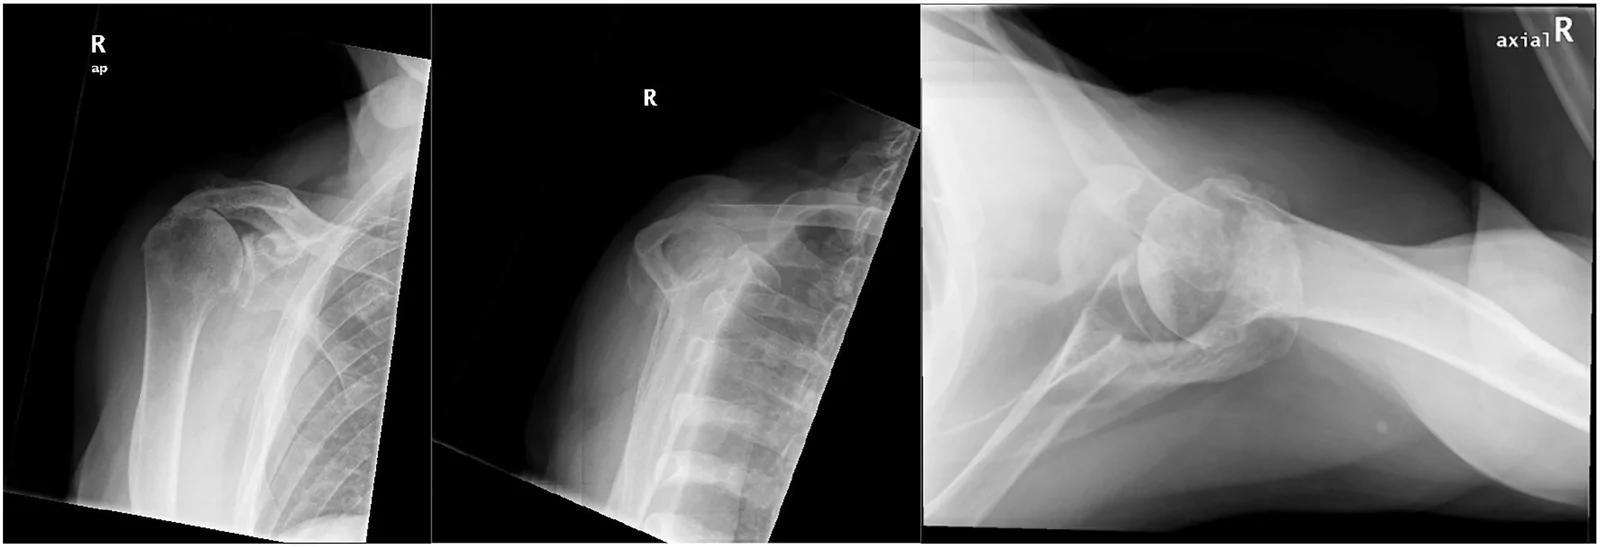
Case 1 pre-operative x-rays showing severe cuff tear arthropathy.
